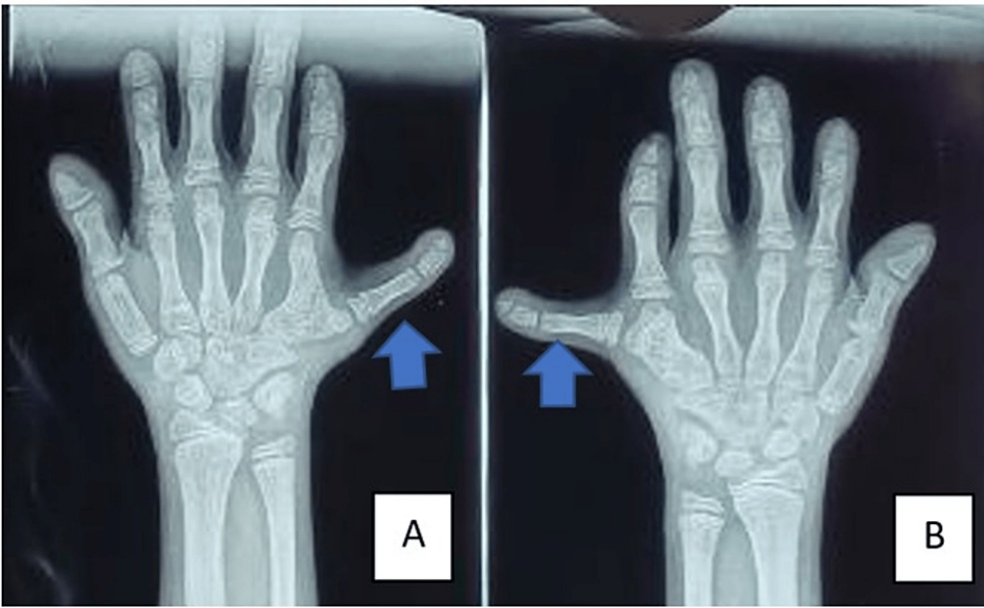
X-ray of bilateral hands: A) Polydactyly in the right hand (blue arrow). B) Polydactyly in the left hand (blue arrow).
